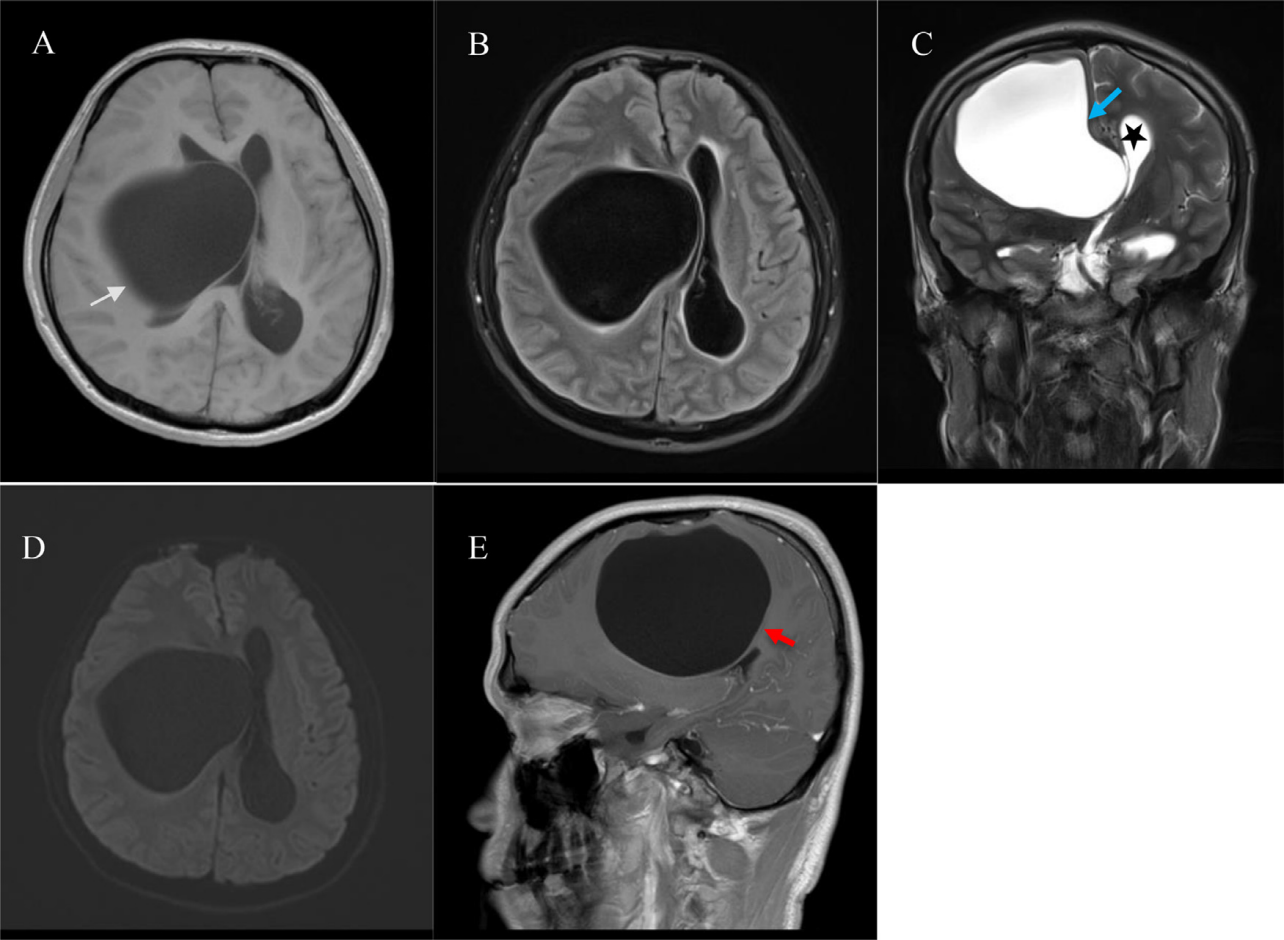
T1-weighted axial (A) plane and FLAIR (B) image showed the cyst with a well-defined thin wall in low signal (arrows). T2-weighted coronal (C) plane showed high signal intensity (blue arrows), compressing the interventricular foramen responsible for hydrocephalus (star). (D) Diffusion-weighted imaging (DWI) showed unrestricted lesions. T1-weighted sagittal plane (E) with contrast media injection showed no enhancement (red arrows).
